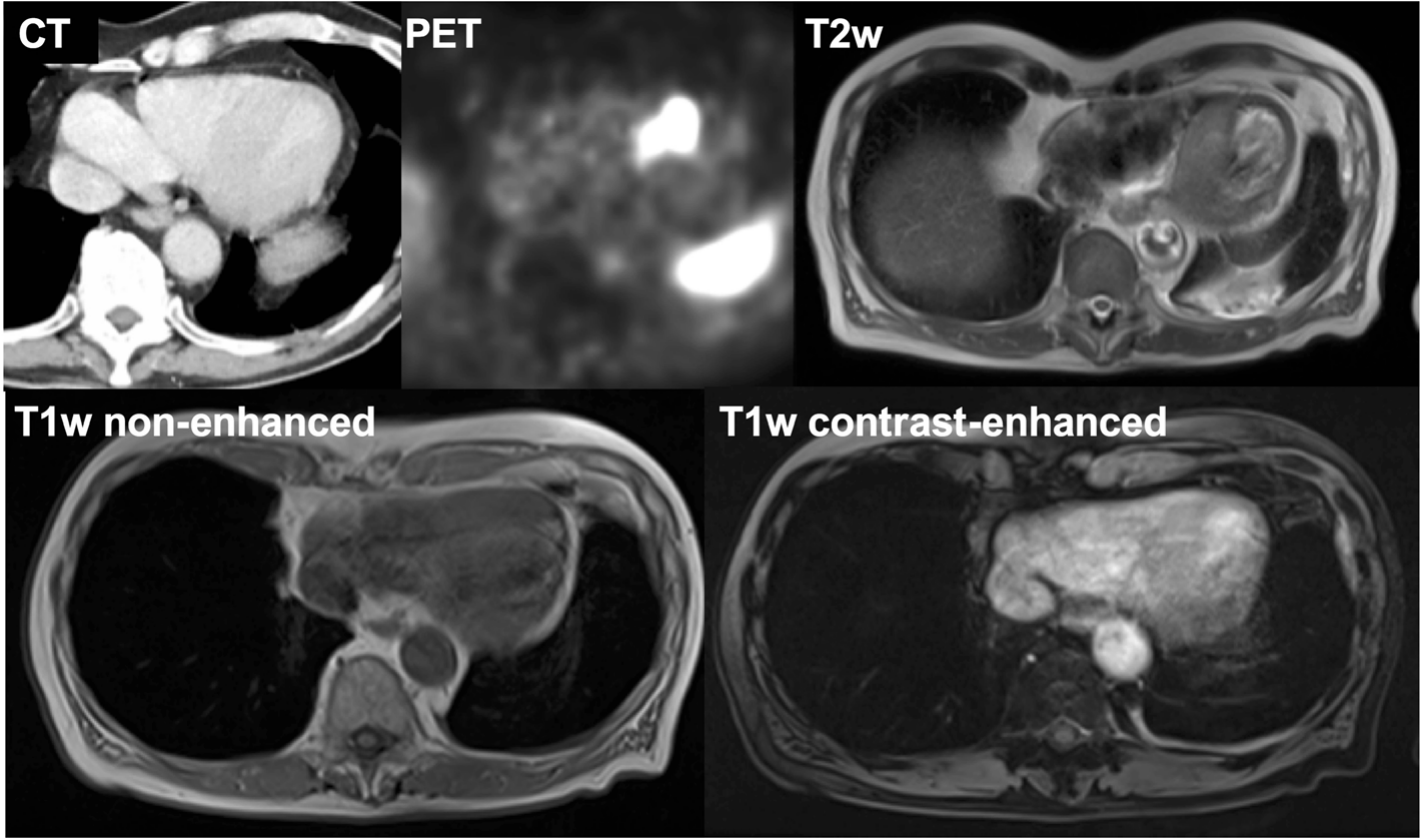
Appearance of a myocardial metastasis on liver MRI performed as part of the complementary staging of the NET disease. A 72-year-old male patient with a G1 neuroendocrine tumor of the small intestine demonstrates a myocardial metastasis in the interventricular septum (evident on CT imaging) with strong 68Ga-DOTATATE tracer uptake in the PET image. The morphologic appearance in the complementary liver MRI is characterized by an intermediate T2w signal and isointense signal on non-enhanced and contrast-enhanced T1w images
